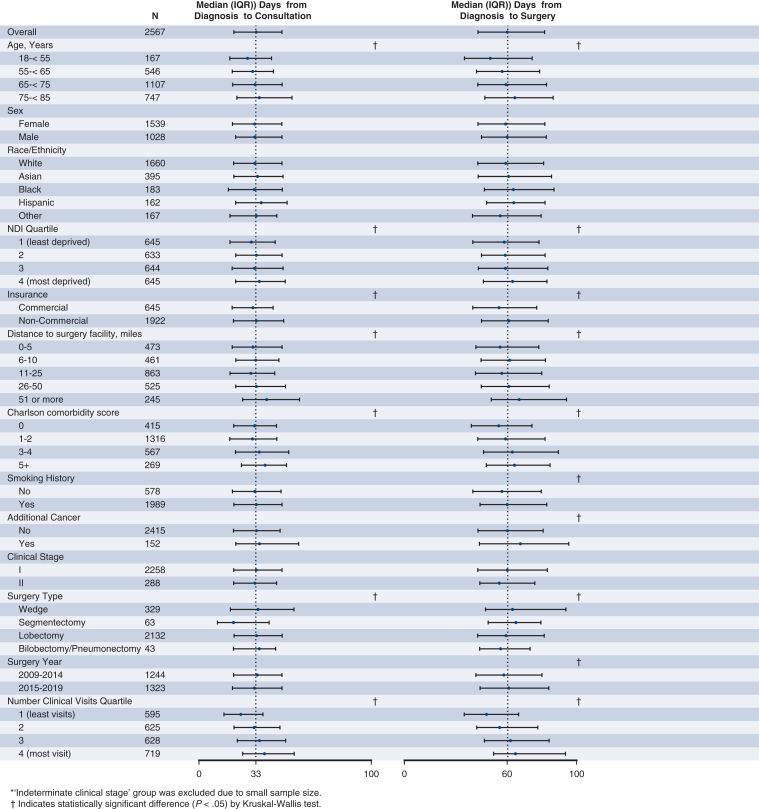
Median time from diagnosis to individual step completion by patient-level factors. *IQR*, Interquartile range; *PET*, positron emission tomography; *CT*, computed tomography; *PFT*, pulmonary function text; *NDI*, Neighborhood Deprivation Index.
